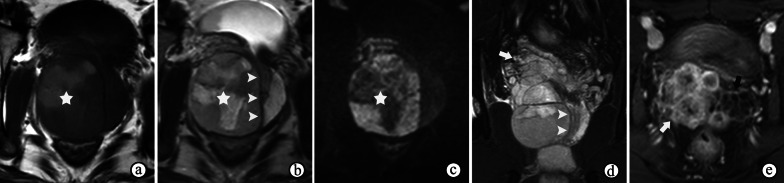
Synovial sarcoma of the prostate in a 26-year-old man, with a serum prostate-specific antigen level of 1.14 ng/mL. a, b T1-and T2-weighted imaging show a heterogeneous signal mass compressing the prostatic urethra and the left lobe of the prostate, with an incomplete, low T2 signal intensity pseudocapsule (arrowheads on b) and irregular areas of hemorrhagic and necrotic changes (☆). c DWI at a b-value of 1200 s/mm^2^ shows a high signal intensity of the solid area and low signal intensity of the cystic area of the mass (☆). d Coronal fat-suppressed T2WI shows the mass compressing the prostatic urethra and the left lobe of the prostate (arrowheads), compressing and invading the right seminal vesicle (arrow). e DCE imaging shows heterogeneous enhancement of the lobulated mass invading seminal vesicles (white arrow). Note the residual tissue of the left seminal vesicle (black arrow)
